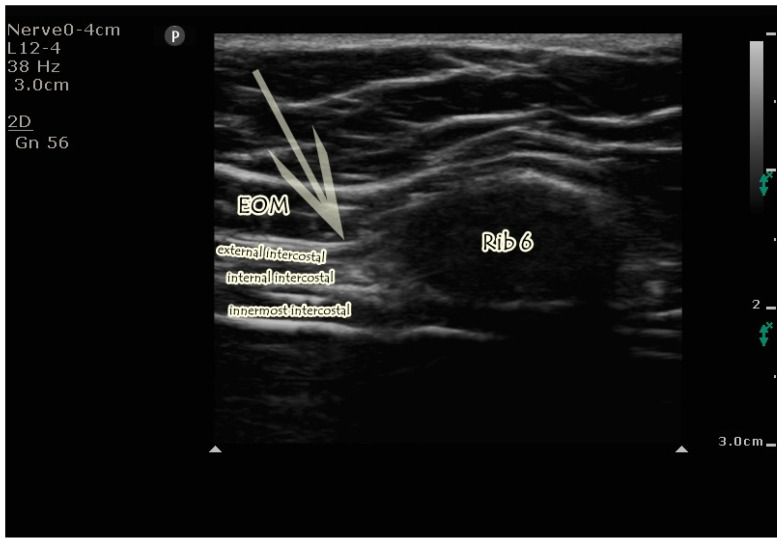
Sonographic visualization of EOIB. External oblique muscle, intercostal muscles, and the sixth rib are seen. The arrow indicates the needle trajectory. The tip of the arrow is between the external oblique muscle and intercostal muscles. EOM; External oblique muscle.
